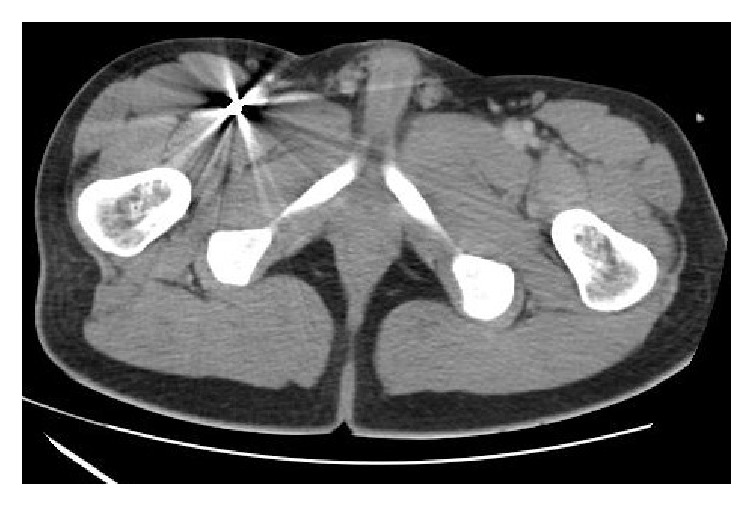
24 hrs after admission, body CT shows the bullet at division of femoris artery.
